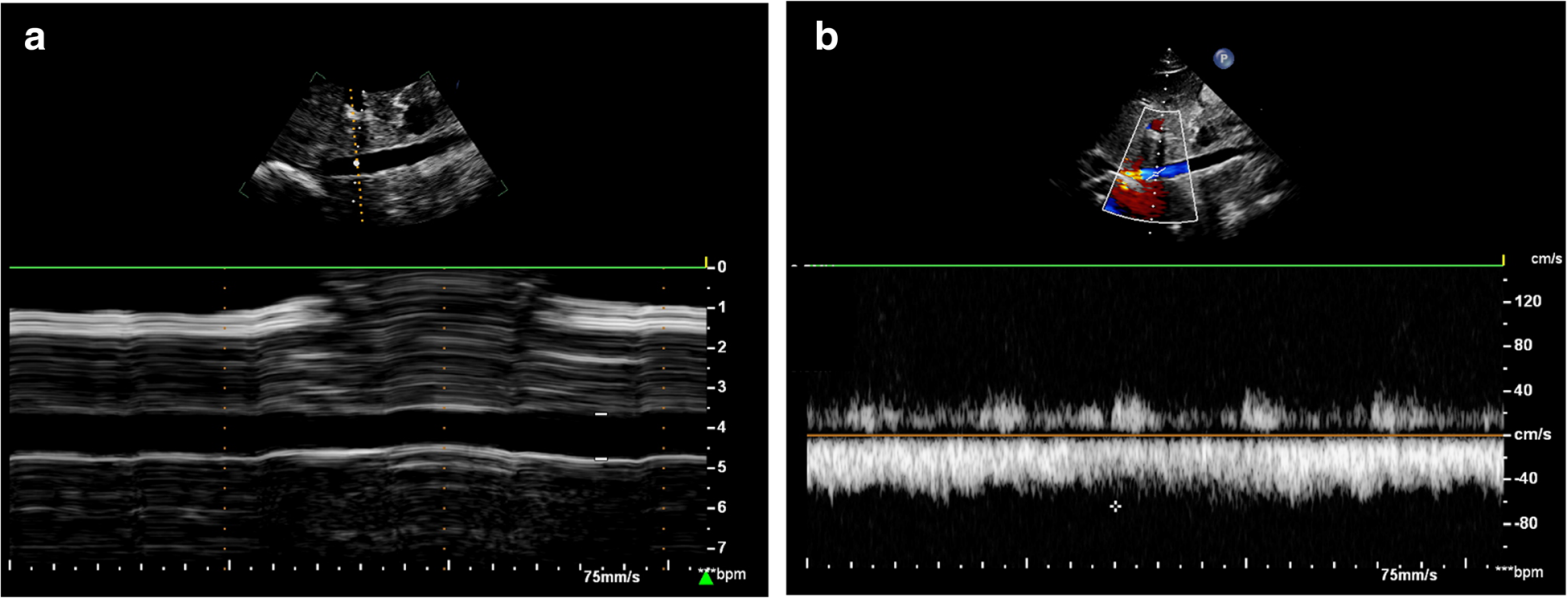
Measurement of IVC by ultrasound with the cardiac probe. a M-mode image showing the anteroposterior diameter of IVC; b Pulsed-wave Doppler-mode image showing the peak velocity of IVC; IVC, inferior vena cava
